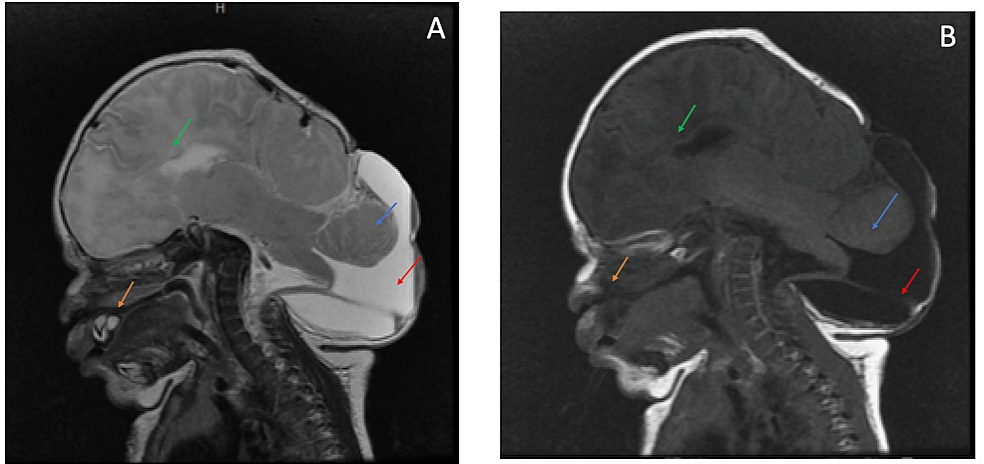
(A) MRI T2 (sagittal view). (B) MRI T1 (sagittal view). Both images show the low occipital/high cervical encephalocele. Green arrow: only the genu of the corpus callosum is seen, indicating partial agenesis. Blue arrow shows the brainstem in the encephalocele. Red arrow shows the hydrocele. Orange arrow shows the palate cleft defect. The midbrain is abnormal as well.
